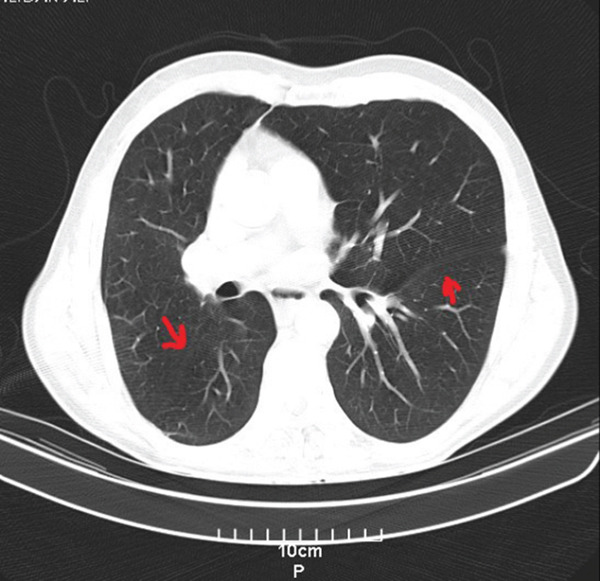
Chest CT scan, dispositioning of the right fissure.
